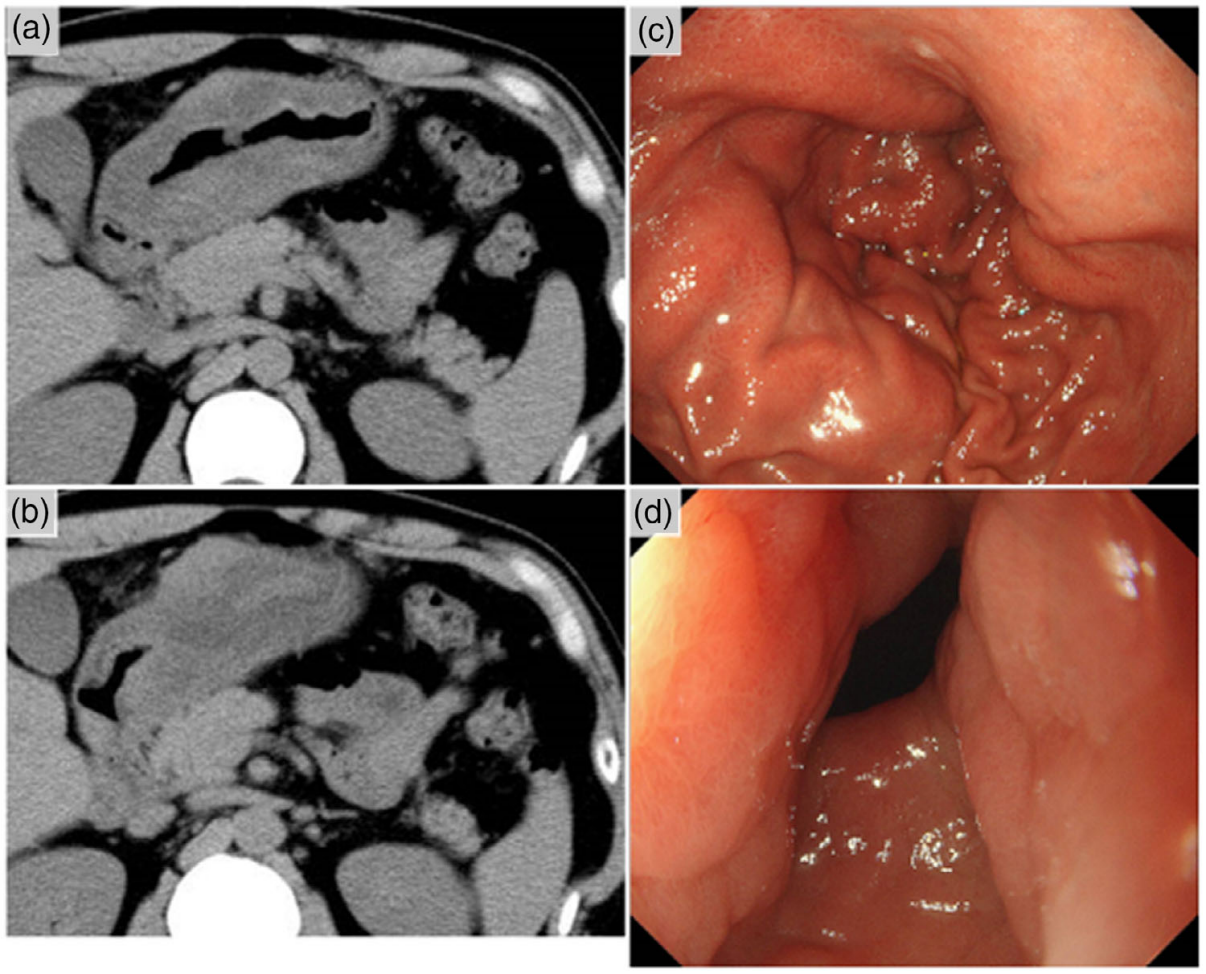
Imaging findings at the initial onset of the pancreatitis of gastric ectopic pancreas. Computed tomography revealed massive thickening of the gastric wall (a,b), and gastroscopy revealed diffuse erythema and edematous thickening of the gastric mucosa (c,d).
